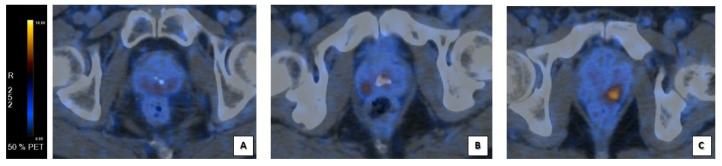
Corresponding visual score at PSMA-PET/CT scans’ analysis. Fusion images of the prostate gland: (A) faint diffuse PSMA uptake at prostate gland in VS1+ corresponding to image D in Figure 1 (B) focal PSMA uptake at the right lobe of prostate’s base in a VS2+ corresponding to image E in Figure 1; (C) focal, intense PSMA uptake at the left lobe of prostate’s base in a VS3+ corresponding to image F in Figure 1.
